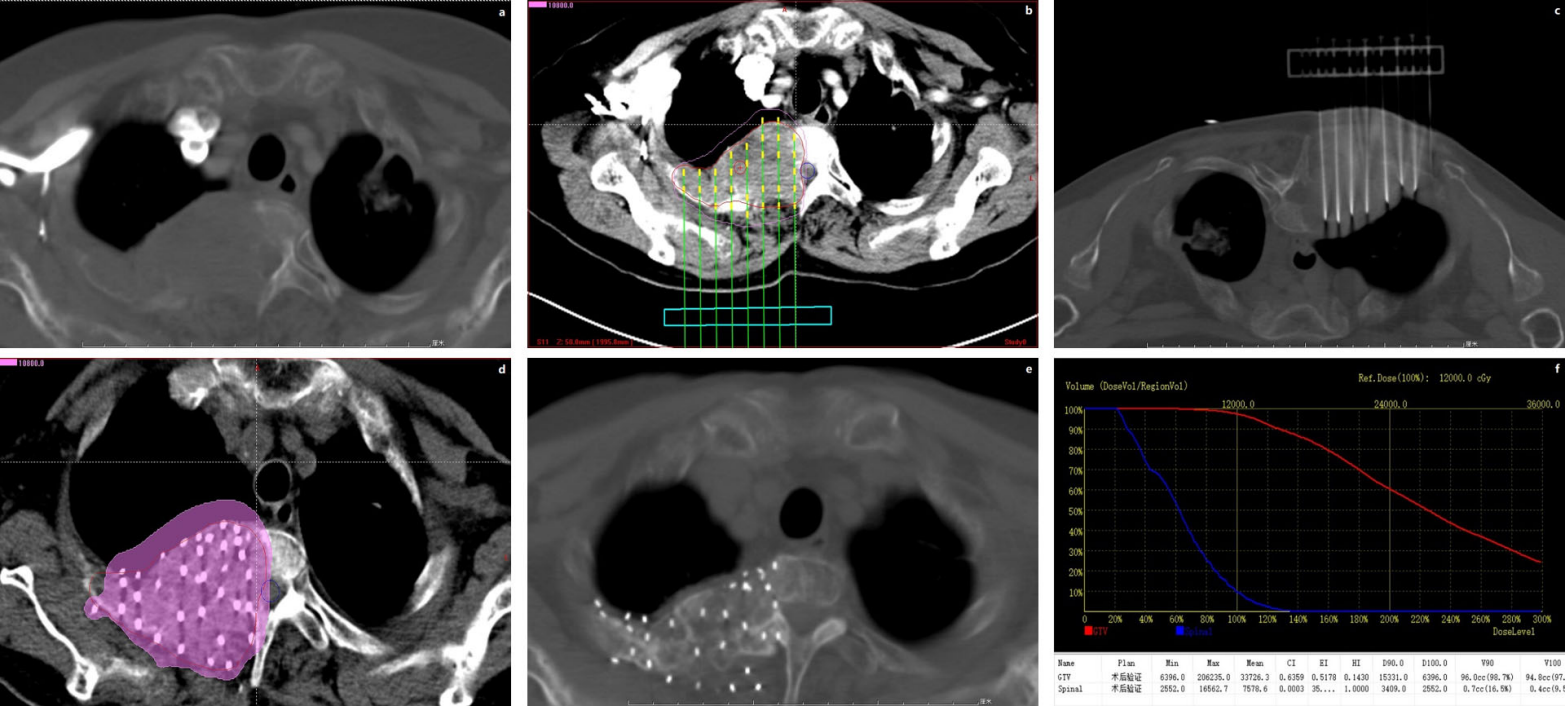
Represent CT scan of a patient during the whole treatment process. (A) Preoparative image. (B) Preoparative plan. (C) Operation process. (D) Postoperative Dosimetry Evaluation. (E) Twelve months after ^125^I seed implantation. (F) Postoperative Dose Volume Histogram.
